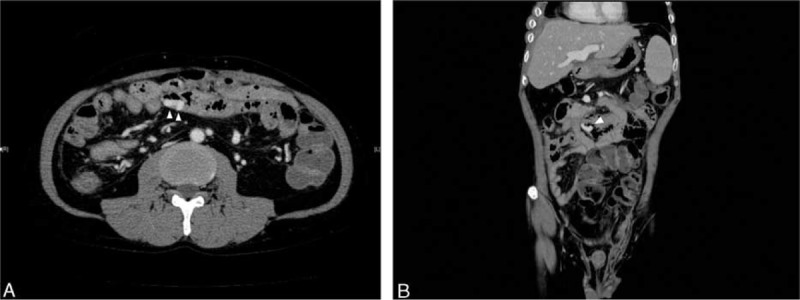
The images of dynamic multidetector computed tomography on admission; the varices protruding into jejunal lumen is pointed by white arrow heads.
